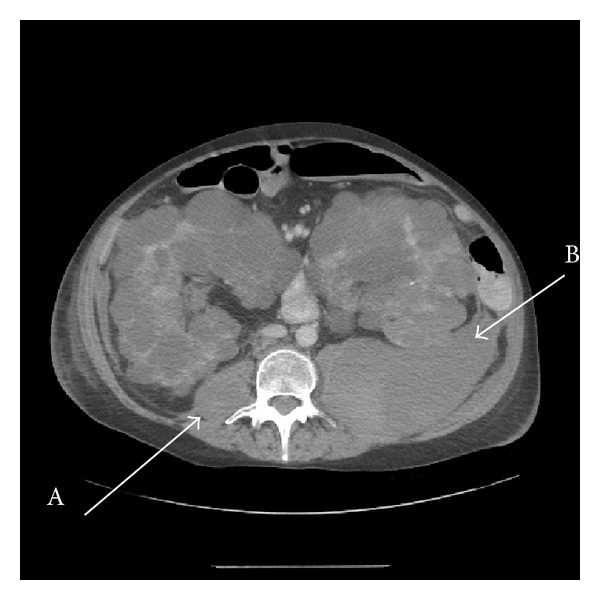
CT scan showing extensive retroperitoneal haemorrhage involving both psoas muscles (left (B) more than right (A) at this level).
